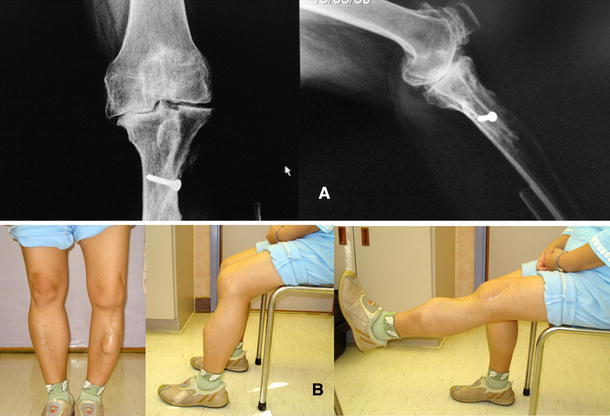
Twenty-five years result. a X-rays showing tri-compartmental osteoarthritis of the knee joint. b Clinical photographs at 25 years
